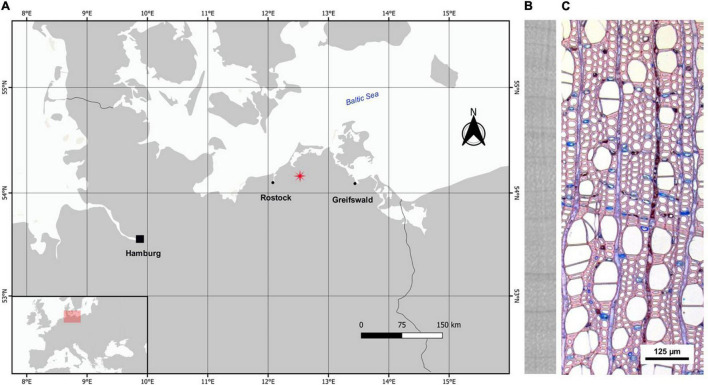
(A) Study area in NE Germany, (B) x-radiography of alder wood, (C) thin section of alder stem for xylem anatomical analysis. Vessels, fibers, ray and axial parenchyma, and a ring boundary (in the middle) are visible in the section, which belongs to tree ADAG01 from the drained stand.
